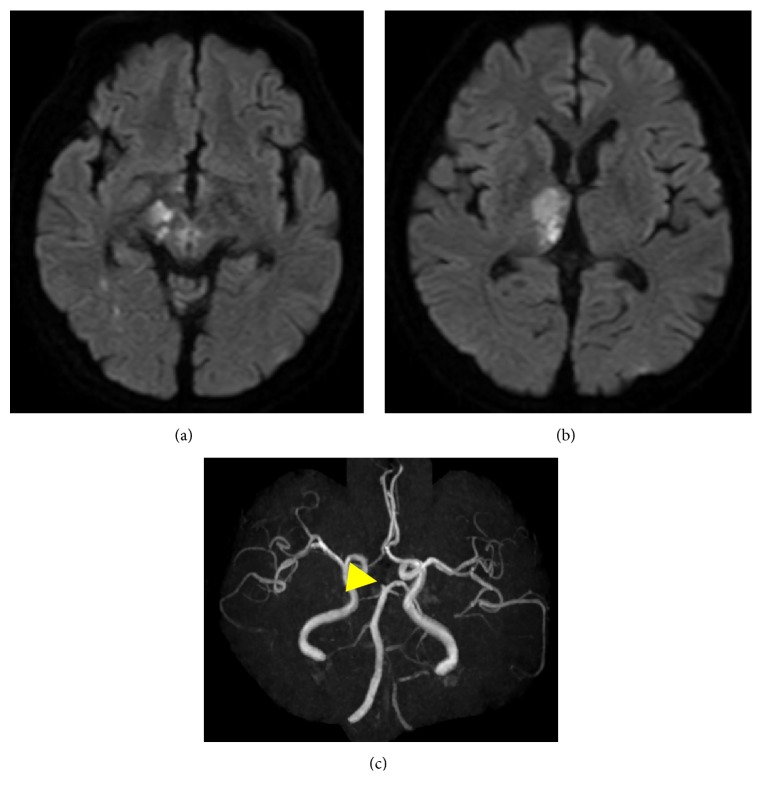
Head MRI performed after aggravation of neurological symptoms showed enlargement of infarction (a, b). MRA showed extension of occlusion (arrow head) to the P1 (c).
